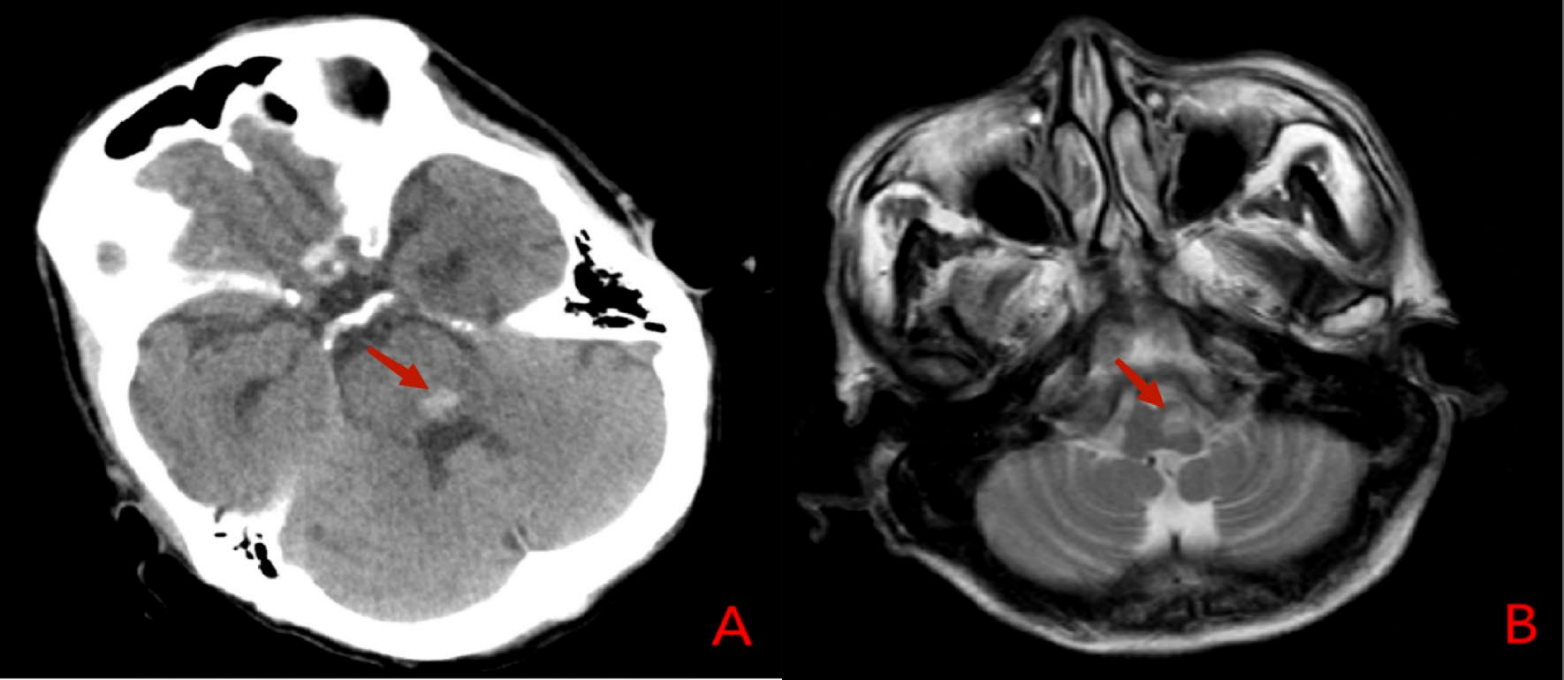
Case No.1, Head computed tomography (A) revealed pontine hemorrhage on the left side; T2WI (B) revealed hyperintensity on the ipsilateral ION 4 months after the insult.
